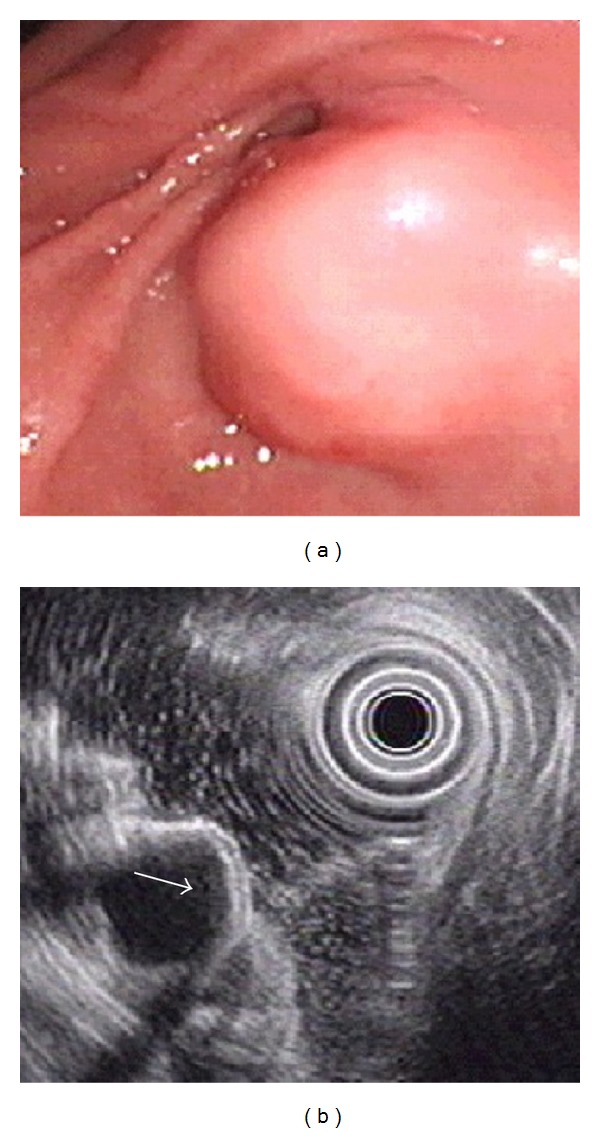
Ectopic pancreas originates from the fourth sonographic layers of the gastric wall. (a) A subepithelial tumor with intact mucosa was identified at antrum. (b) Endoscopic ultrasonographic image obtained with a 12 MHz catheter probe. The tumor exhibits homogenous, hypoechoic echogenicity and distinct margin originating from the fourth sonographic layer of gastric wall (arrow).
